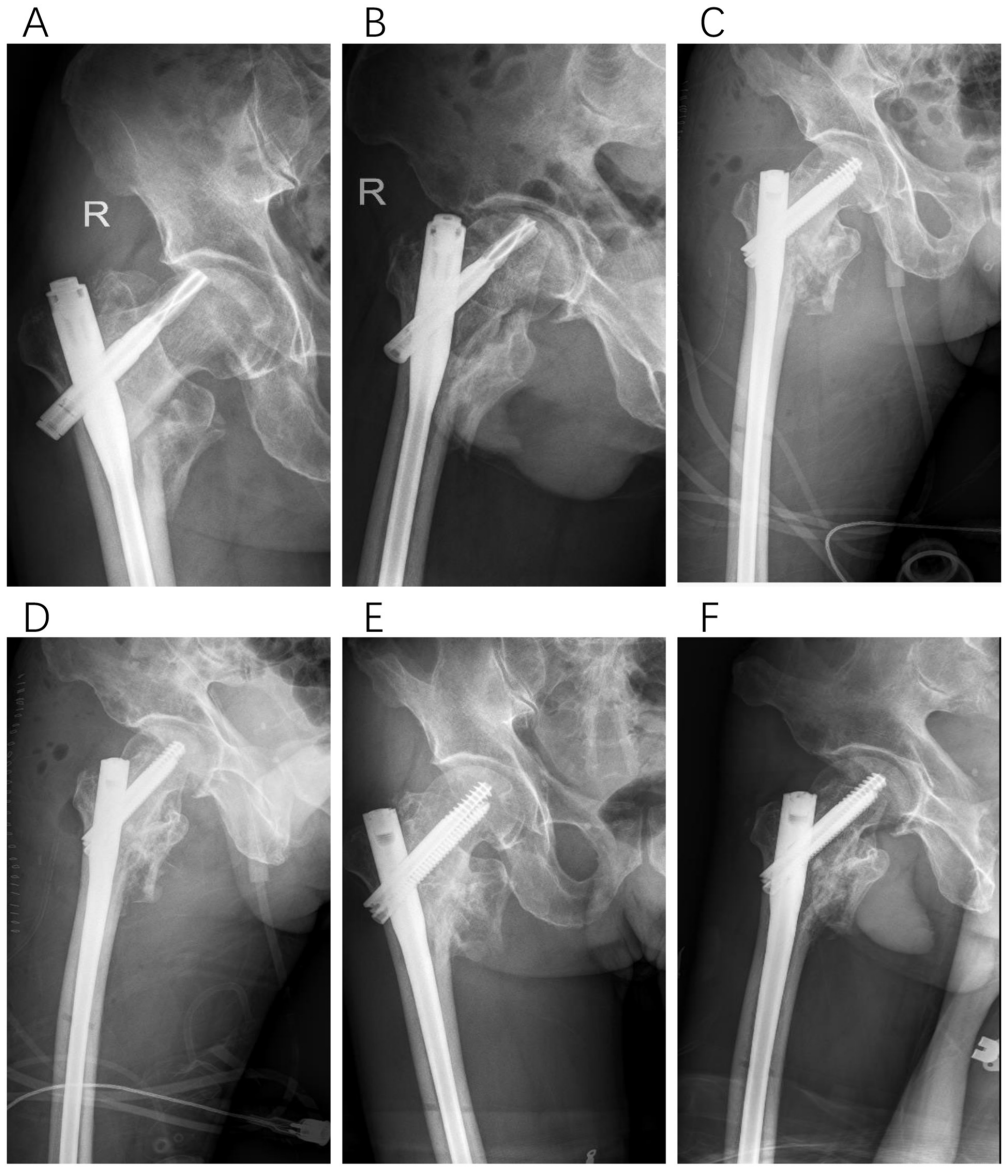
Type II-C, a 62-year-old female patient underwent PFNA fixation for a right intertrochanteric femoral fracture. Seven months post-operatively, radiographic evaluation revealed screw cutout through the femoral head. During the subsequent revision surgery, considering the relatively young age of the patient and minimal damage to the femoral head, we performed reduction, artificial bone grafting, and internal fixation with intramedullary nails. Successful bony union was achieved 6 months following this revision. (A, B) Anteroposterior and lateral X-rays of the femur obtained 7 months after initial PFNA fixation. (C, D) Anteroposterior and lateral X-rays of the femur immediately following the revision surgery. (E, F) Successful bony union confirmed 6 months post-revision surgery.
